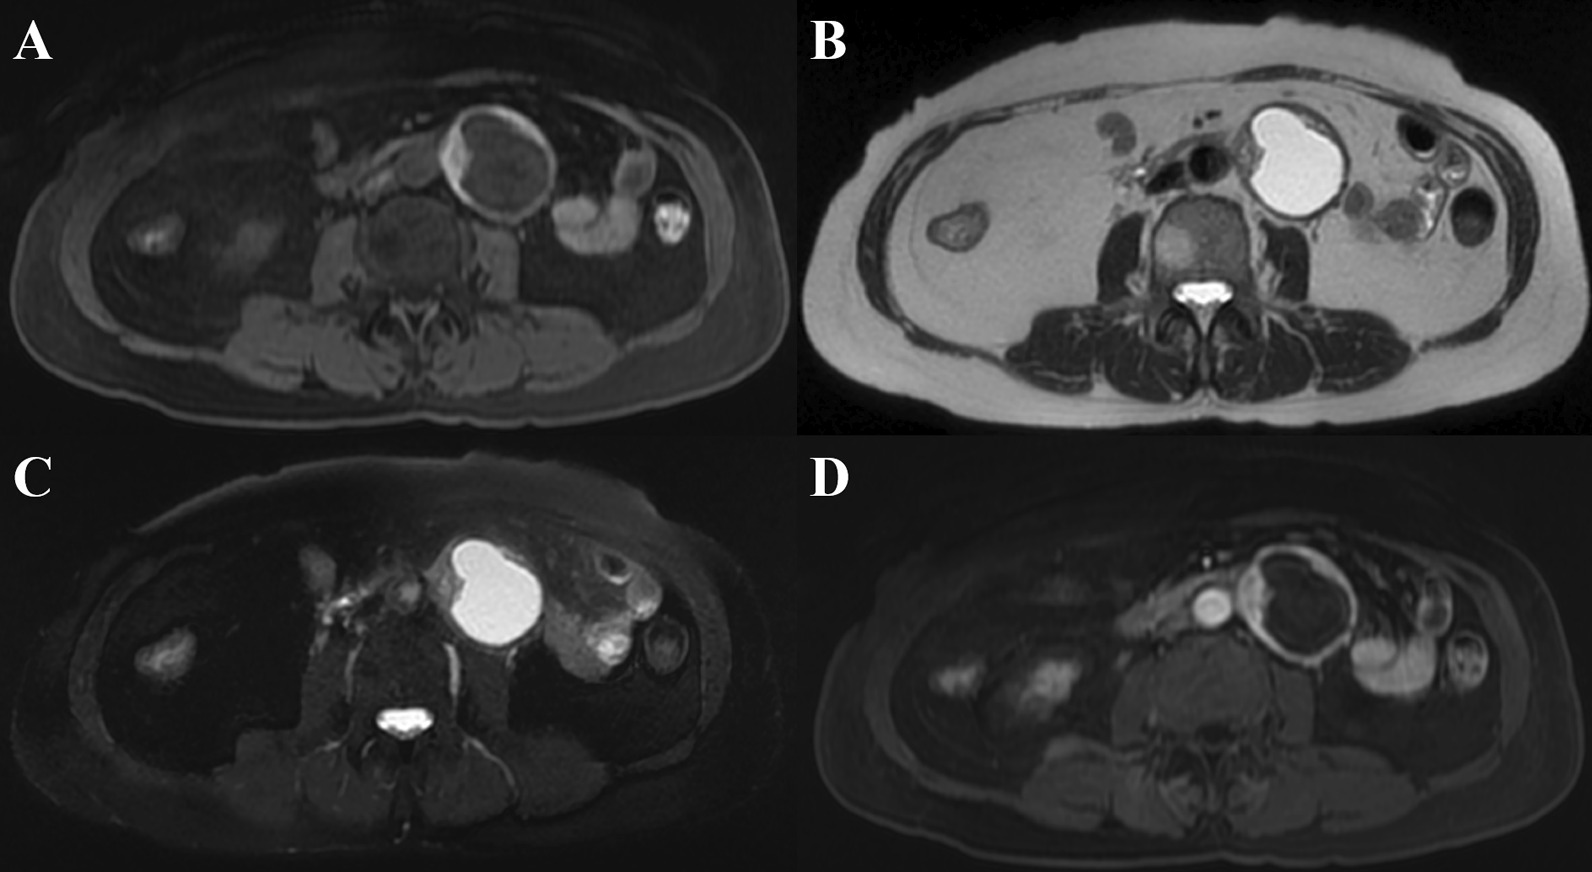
Contrast-enhanced axial MRI scans in a 59-year-old woman with MMNST. A A 4.7 cm cystic mass at the para-aortic region, abutting onto the 3rd and 4th portion of the duodenum, showed a hyperintense solid part and a hypointense cystic part on T1WI, and B a hypointense solid part and a hyperintense cystic part on T2WI. C T2WI with fat suppression also showed a hypointense solid part and a hyperintense cystic part. D Contrast-enhanced T1WI showed identical enhancement with T1WI
